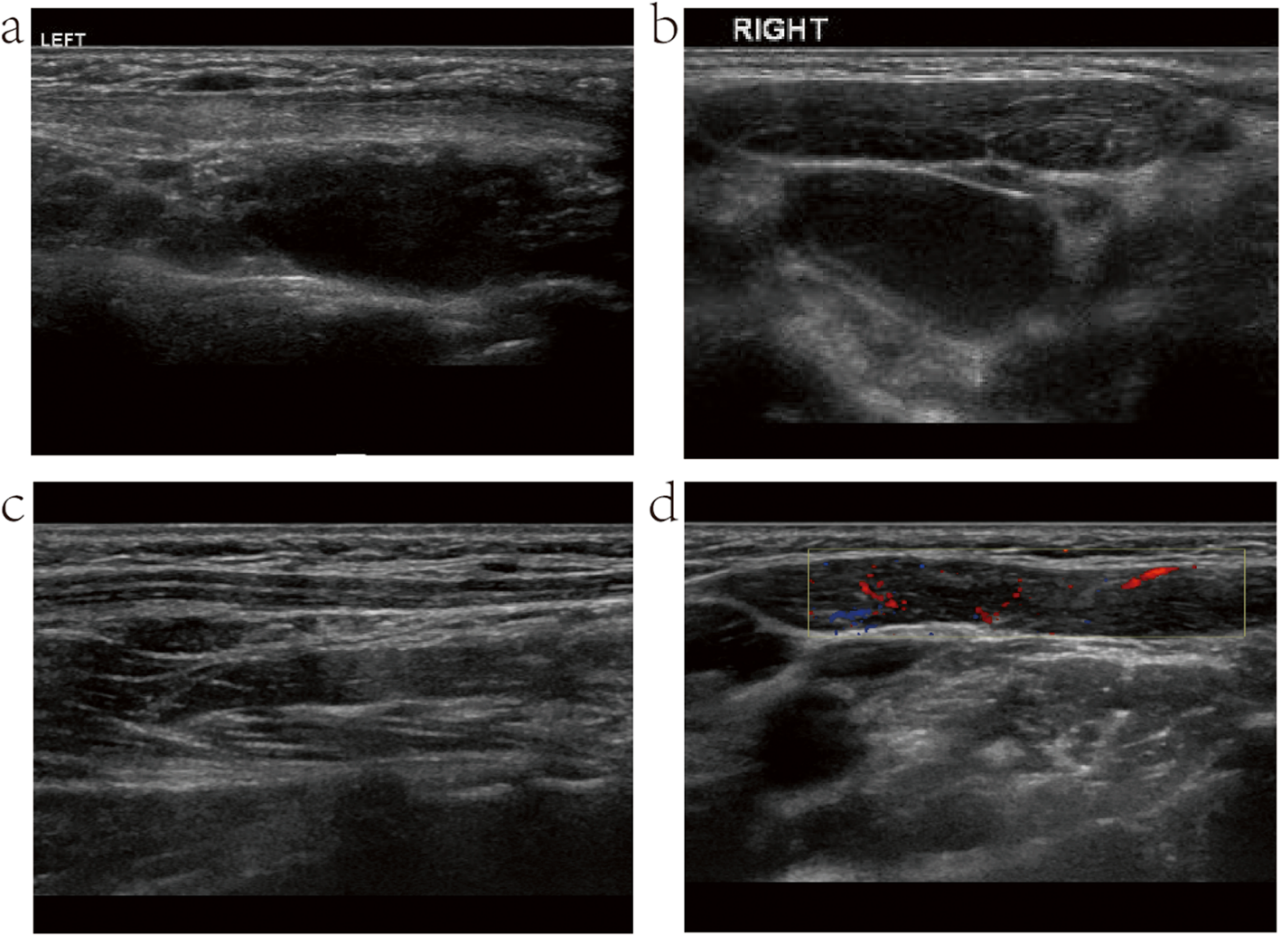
Ultrasound images of the two children in Case one and Case two at final follow-up (a) and (b) Ultrasound images of the involved and normal SCMs of the child in Case one at final follow-up, respectively, showing amyotrophy of the involved SCM (c) and (d) Ultrasound images of the involved and normal SCMs of the child in Case two at final follow-up, respectively, showing amyotrophy of the involved SCM
